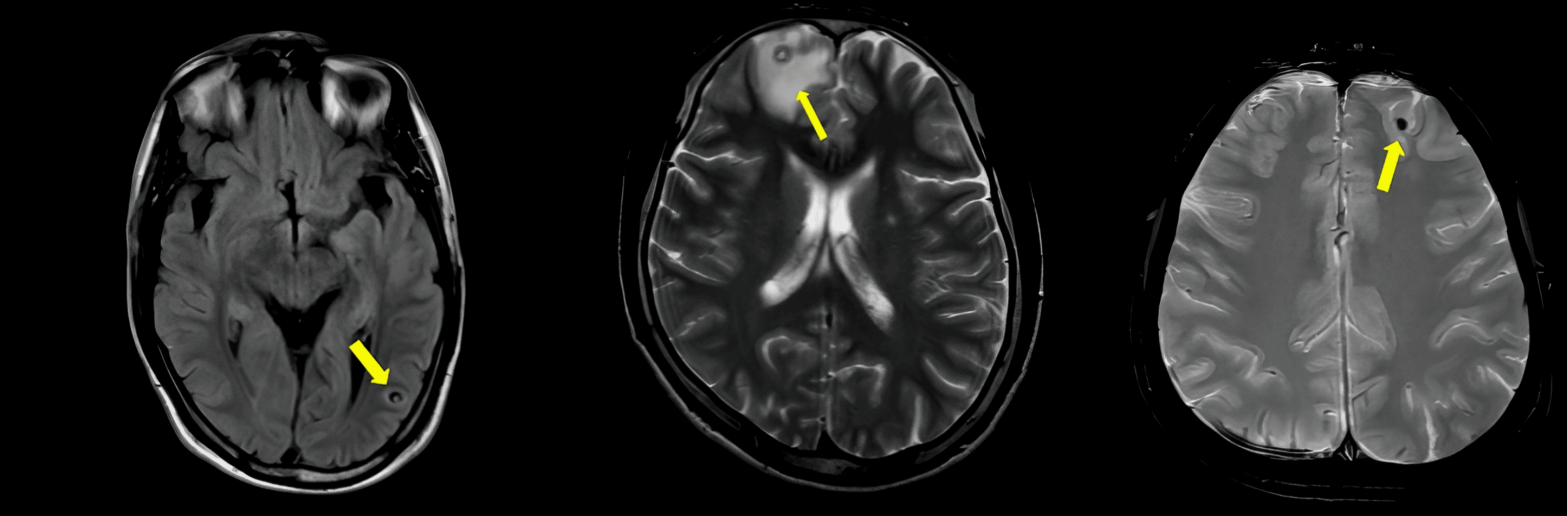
Brain MRI images of NCC in different stages: Vesicular stage (Left), Colloidal vesicular stage (Middle), and Calcified stage (Right).
